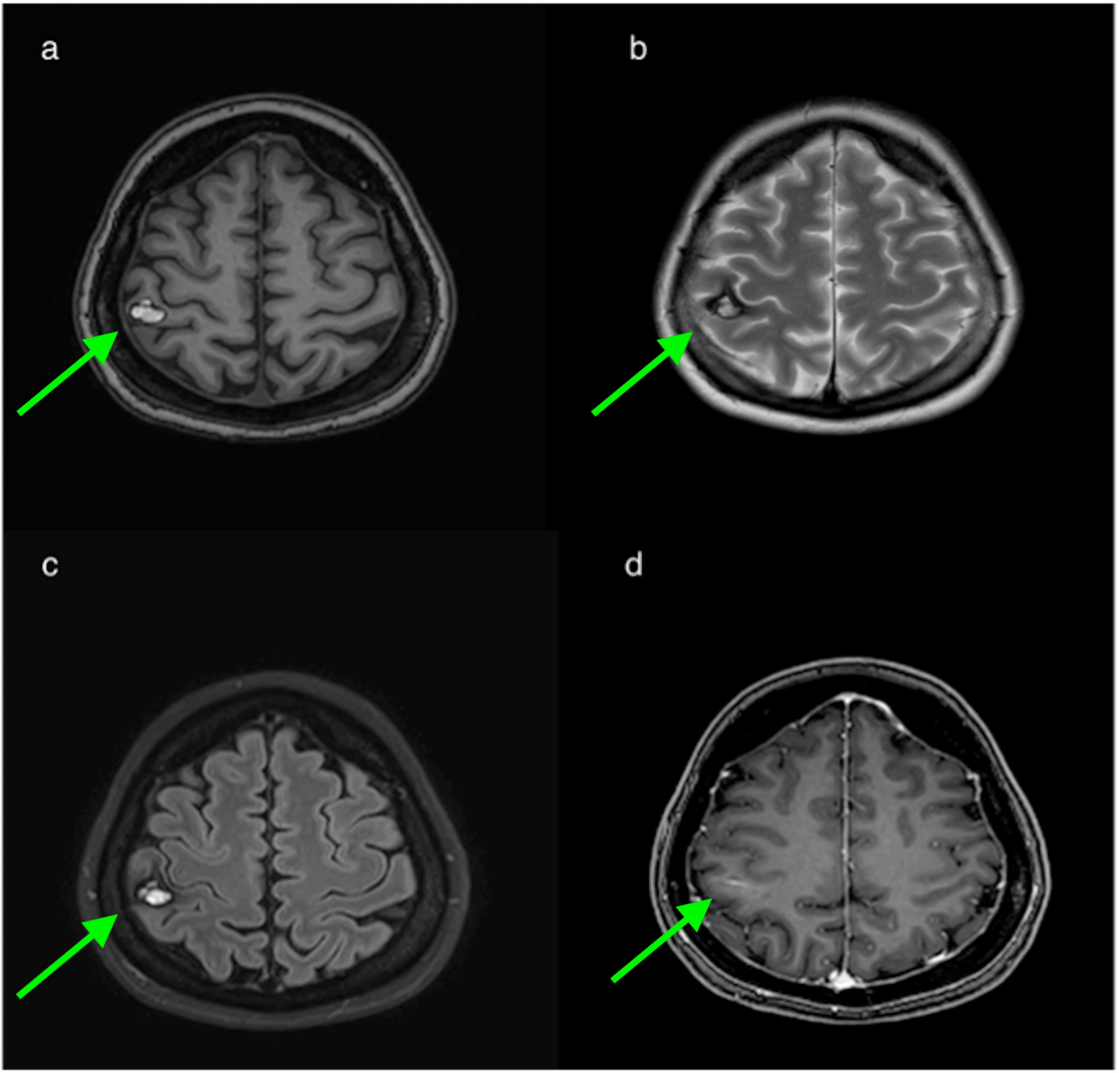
Follow-up MRI images On follow-up MRI images taken two months later, the center of the lesion is hyperintense on T1WI (a), T2WI (b), and FLAIR (c) sequences, with a low-signal hemosiderin rim on the periphery in T2WI and FLAIR. Additionally, a developmental venous anomaly running immediately inferior to the lesion is visible on the contrast enhanced T1WI sequence (d). T1WI : T1-weighted images; T2WI: T2-weighted images; FLAIR: fluid-attenuated inversion recovery.
